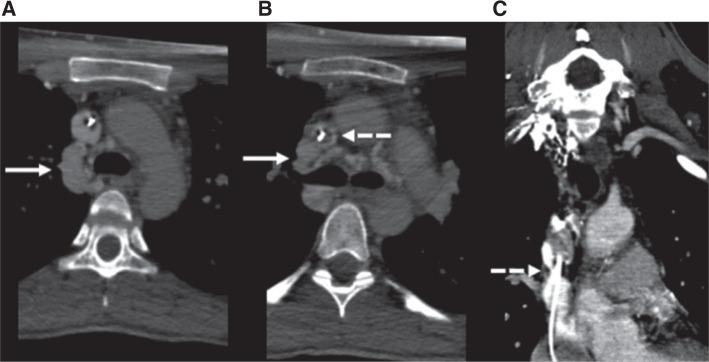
Computed tomography angiography was performed in Argentina. A and B: Transverse slices showing a dilated azygos vein and azygos arch (solid arrow) as well as a stenotic SVC with possible thrombus (dashed arrow). C: Coronal section showing the RV ICD lead coursing through a stenotic SVC (dashed arrow).
